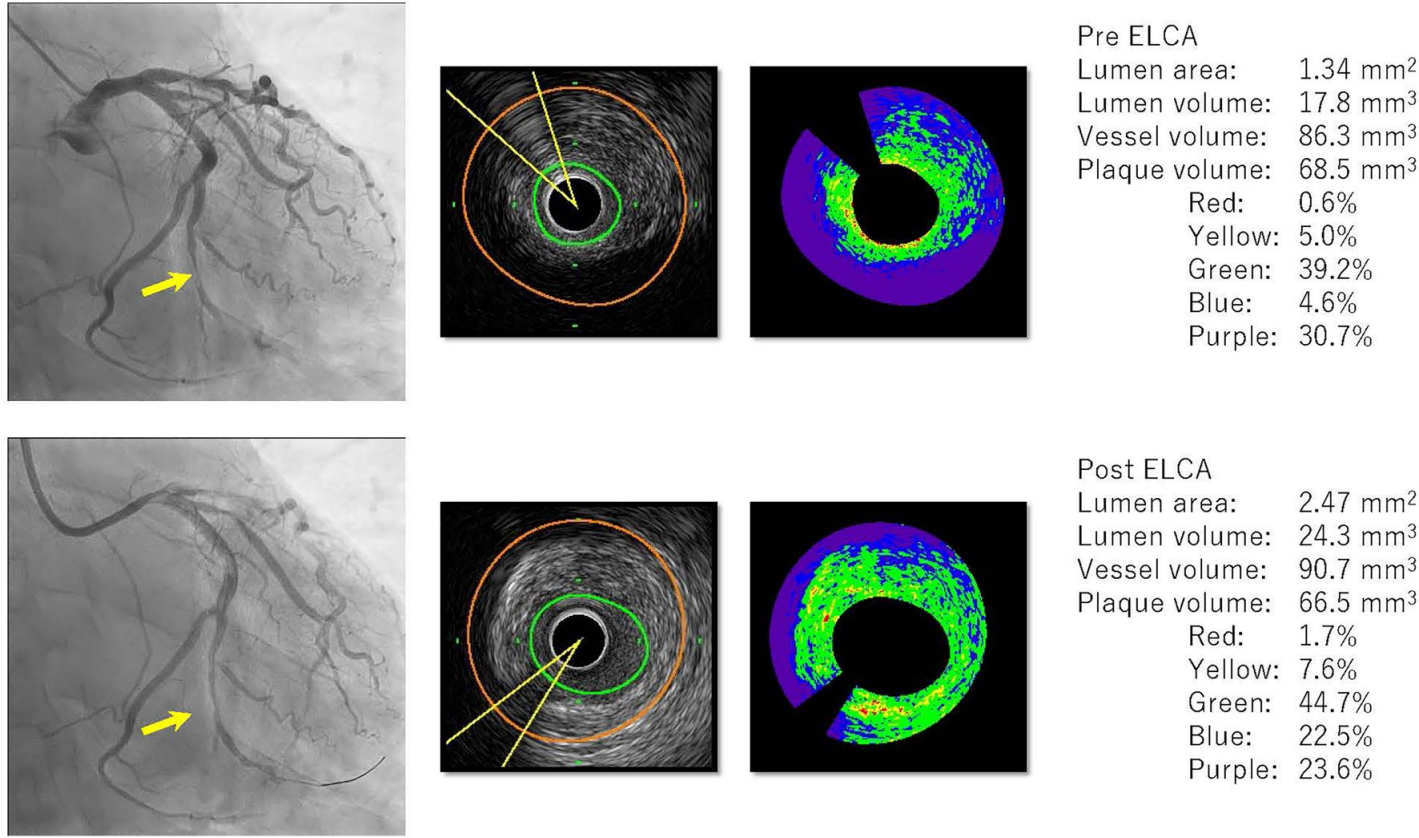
Angiography, conventional IVUS, IB-IVUS images and parameters before and after ELCA of a representative case. Angiography before ELCA (above, left), conventional and IB-IVUS images before ELCA (above middle), IVUS parameters before ELCA (above right). Angiography after ELCA (below, left), conventional and IB-IVUS images after ELCA (below, middle), IVUS parameters after ELCA (below, right). *ELCA* excimer laser coronary angioplasty. *IB-IVUS* integrated backscatter-intravascular ultrasound. *IVUS* intravascular ultrasound
